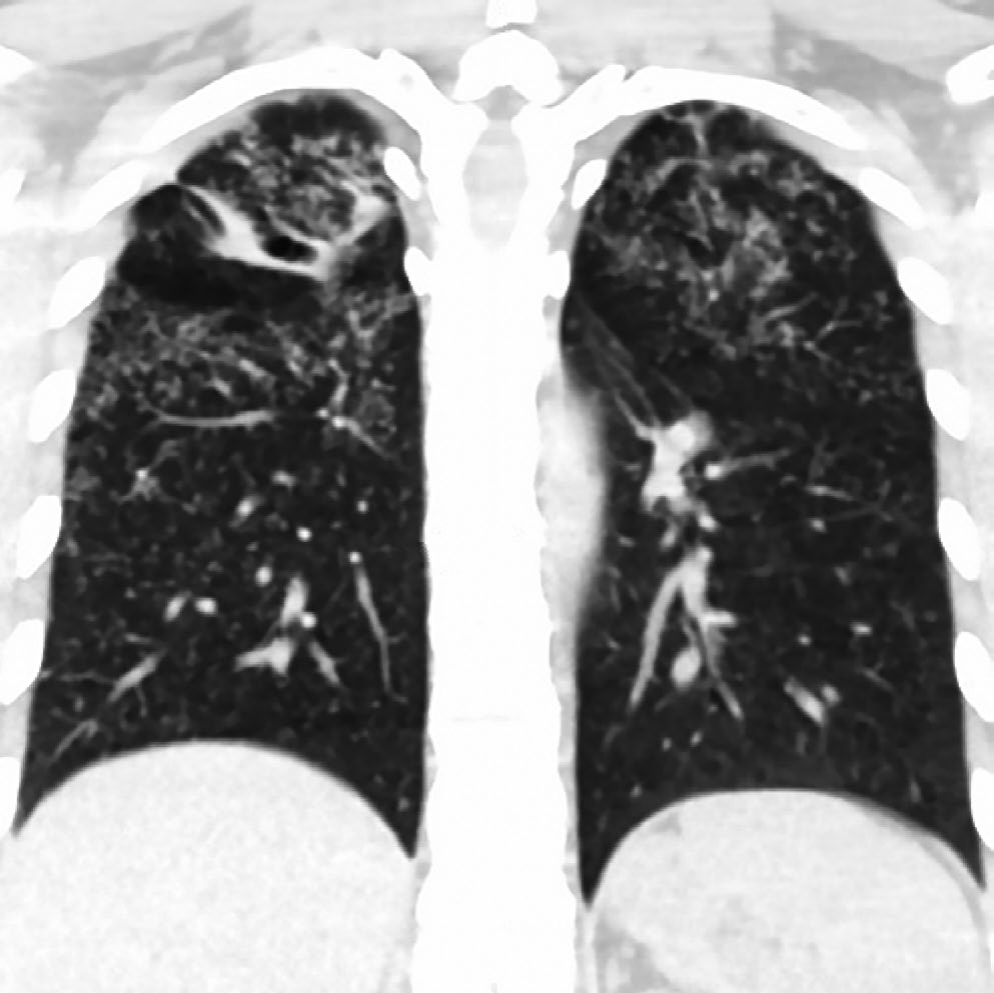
Coronal noncontrast CT image of the chest shows upper lobe predominant infiltrates with a miliary pattern and bronchiectasis positive for mycobacterium tuberculosis in sputum
